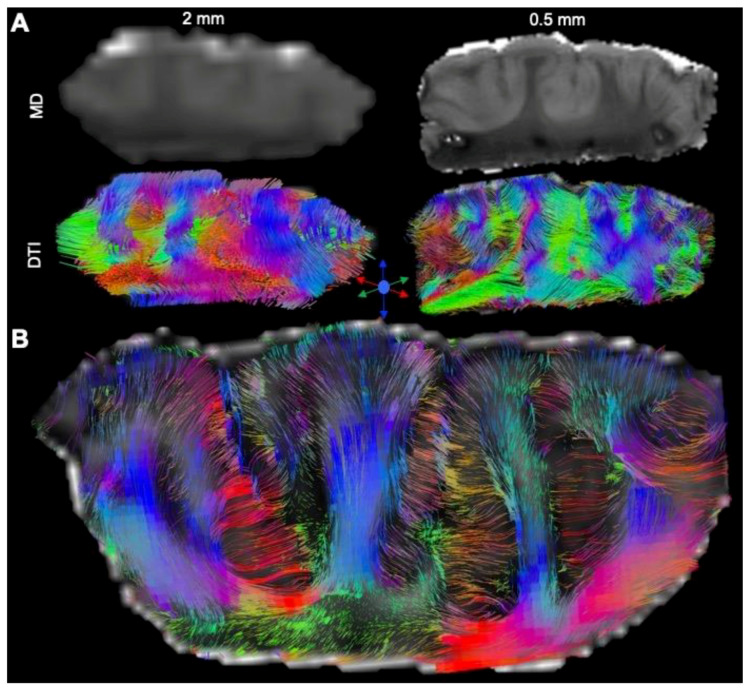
Tractography of cortical dysplasia. (A) Downscaling of mesoscale MR images (0.5 mm isotropic) to a typical clinical resolution (2 mm isotropic) demonstrates the inherent limitations of lower-resolution scans to identify and separate individual folia, as well as partial volume effects that compromise a robust delineation of white and grey matter. Partial volume effects are also evident in the tractography at the clinical resolution, especially in terms of distinguishing streamlines entering the grey matter. (B) In contrast, an isotropic mesoscale resolution provided a robust tractography through the sample in a coronal view. Branches of white matter feeding into individual gyri before fanning out into the cerebral mantle are readily visualized and traced.
